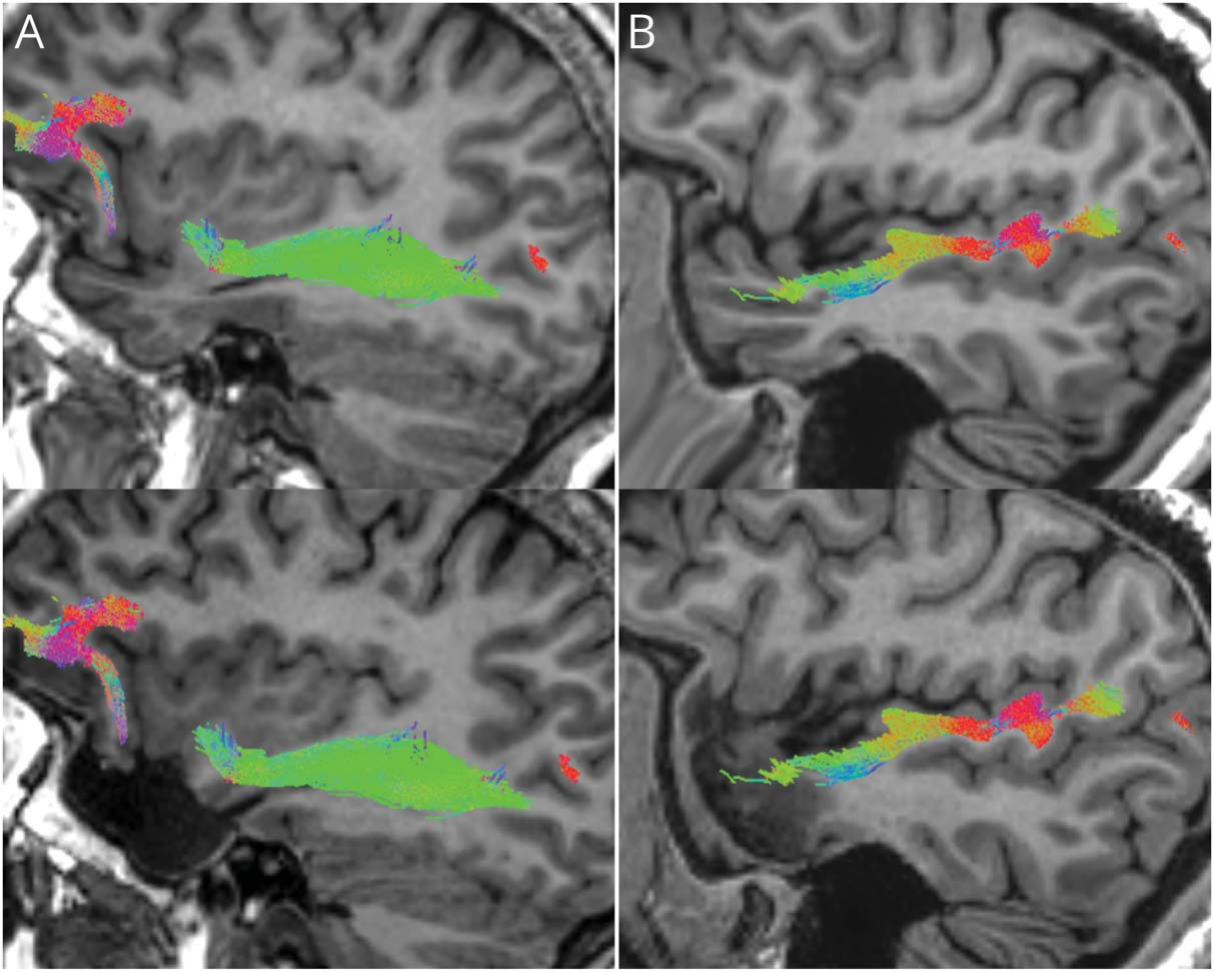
Sagittal Representation of a Patient With the Inferior Fronto-occipital Fasciculus (A) and Middle Longitudinal Fasciculus (B) Spared in Left Anterior Temporal Lobe Resection For each bundle, preoperative (top) and postoperative (bottom) T1-weighted images are shown with tracts overlaid.
